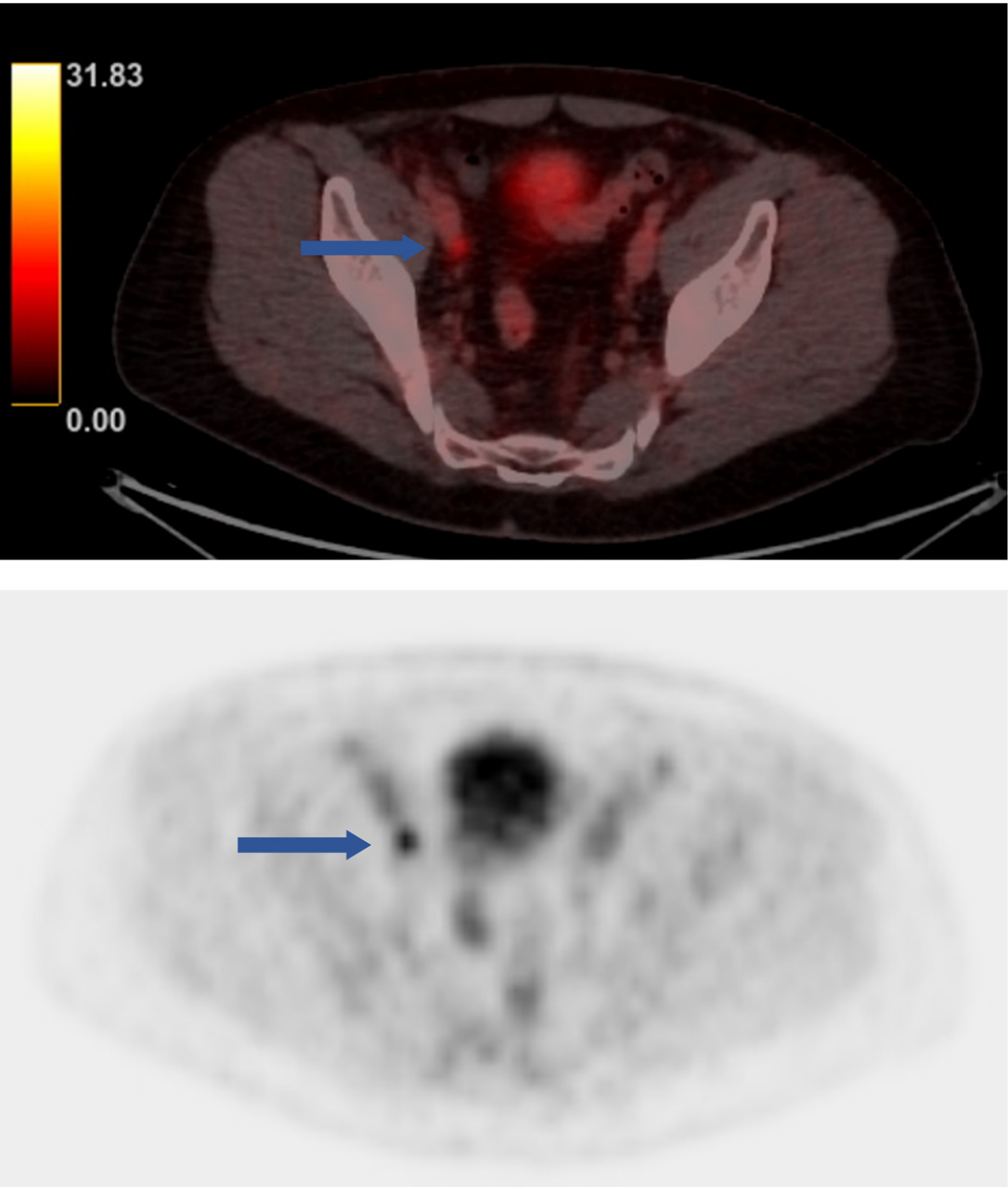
A 66-year-old man with a prostate-specific antigen level of 15 ng/mL was diagnosed with an adenocarcinoma of the prostate. According to international guidelines,^1^ a preoperative ^18^F-rhPSMA-PET/CT^2^ was performed and revealed 2 prostate-specific membrane antigen (PSMA)–avid lymph nodes (LNs), one in the right obturator region (blue arrow) and one in the presacral region (not shown). Radio-guided, robot-assisted extended pelvic LN dissection using a laparoscopic γ-probe was performed 22 hours after IV administration of ^111^In-PSMA-I&T^3^ (NCT04300673). Both LNs were successfully detected and removed in packets.
